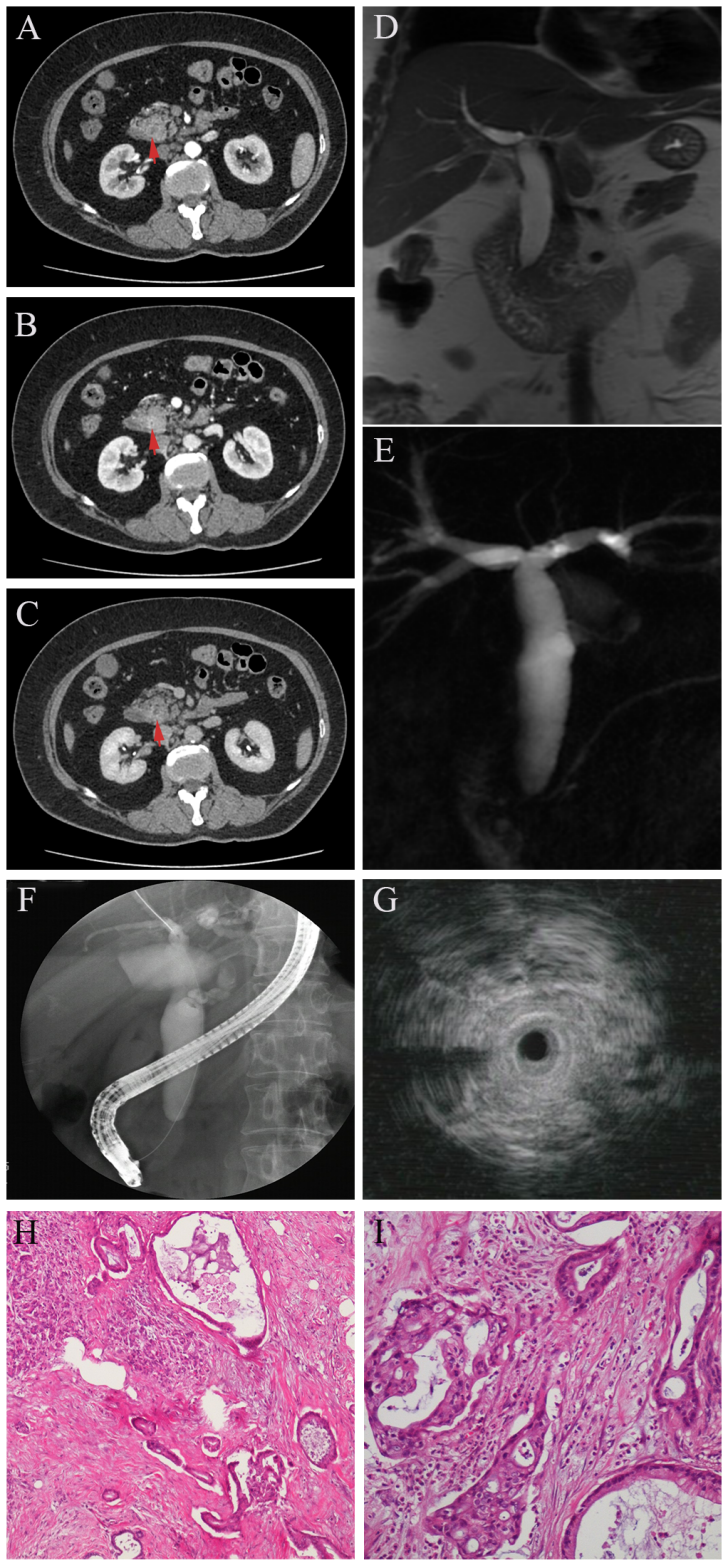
In DCE-CT, the stricture site is not evident in arterial phase (A, red arrow), as well as in delayed phase (C, red arrow), but enhanced in portal phase (B, red arrow). MRCR shows obvious dilation of intra- and extrahepatic bile ducts with distal biliary stricture (D) but a non-dilated pancreatic duct (E). Endoscopic Retrograde Cholangiopancreatography (ERCP) revealed a significant concentric stenosis in the lower common bile duct with significant dilatation of the intra- and extrahepatic bile ducts (F). Intraductal ultrasonography (IDUS) showed thickening of the wall in the stenosis site with destruction of normal lumen structure (G). Postoperative pathology revealed distal biliary stricture due to pancreatic adenocarcinoma (H–I).
